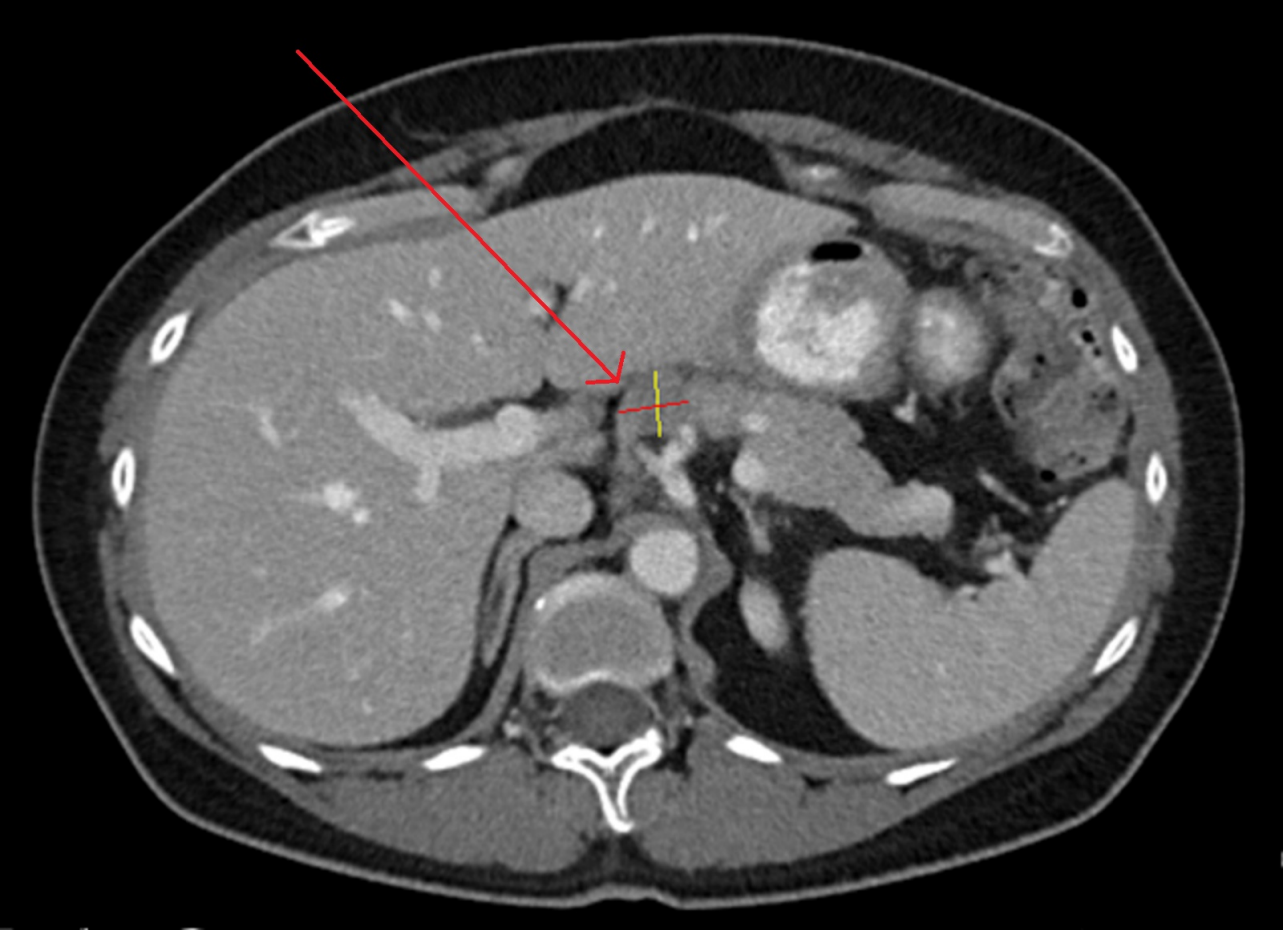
Abdominal CT of Patient 1 after three months of evofosfamide, showing a reduction in size of target lesion to 1.8x1.9 cm, with CA-125 62 U/ml
